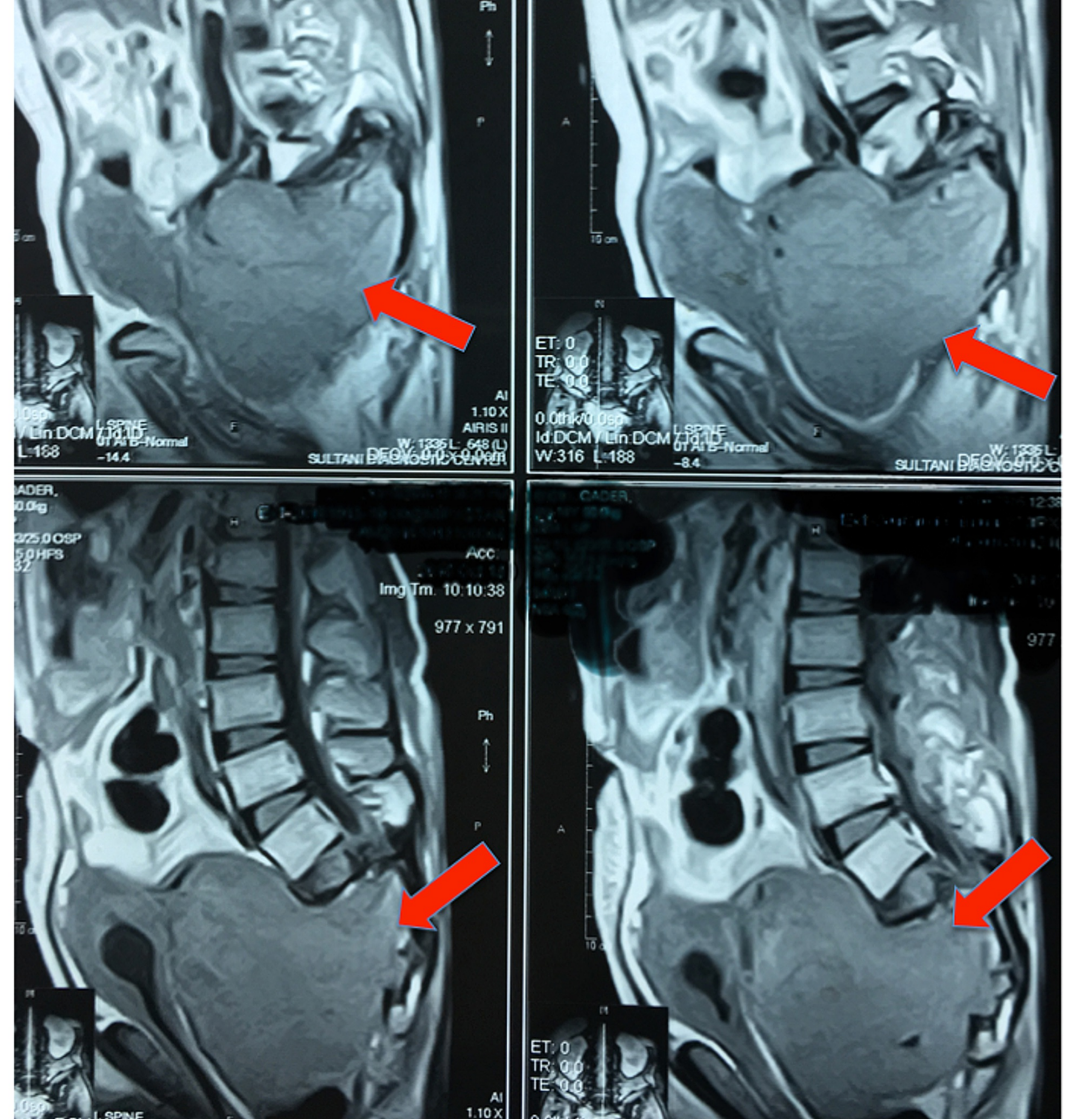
Sagittal MRI image (T1 weighted) of a sacral mass (10.7*10.0*8.3 cm) with near-total destruction of the sacrum, exhibiting local extension The sacral mass projects anteriorly causing forward compression onto the bladder. GCT: Giant Cell Tumor, MRI: Magnetic Resonance Imaging
